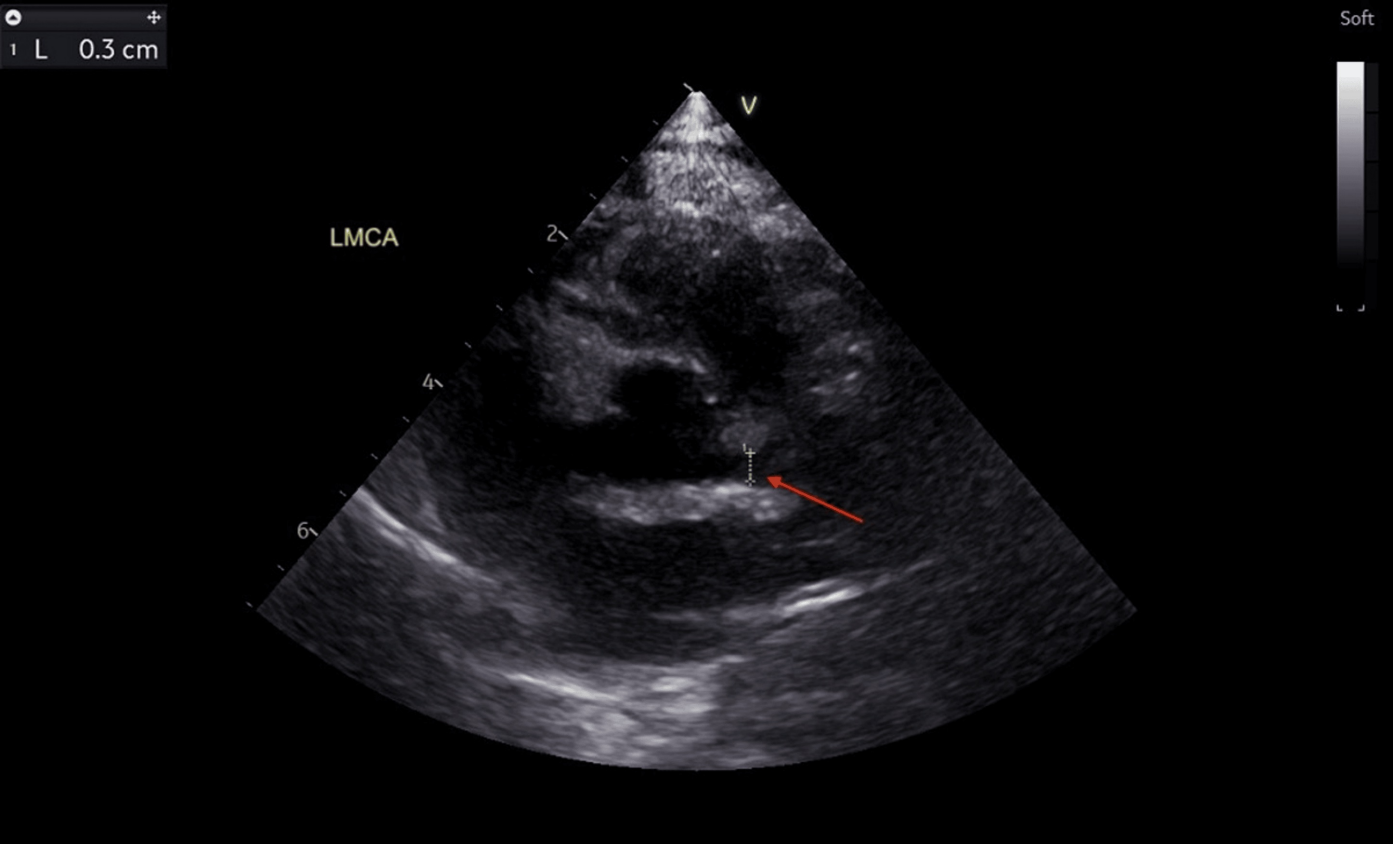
Echocardiogram showing a dilated left main coronary artery. The red arrow points at the dilated left main coronary artery measuring 3.0 mm (Z score: +3.64).
